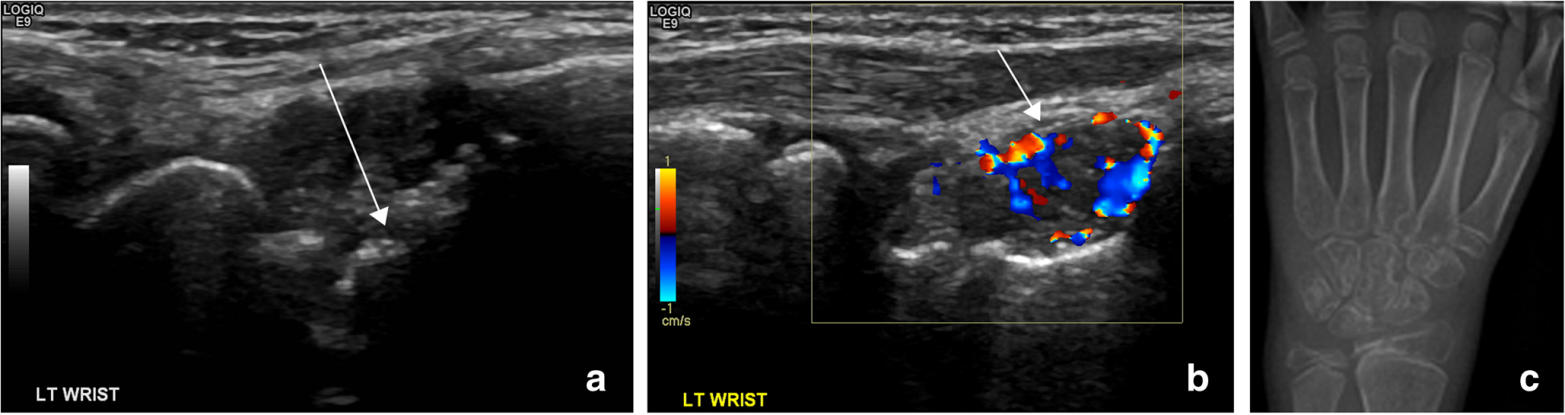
Ultrasound may depict both synovitis and bone destruction, particularly in small joints. a Ultrasound of the dorsal aspect of the wrist shows an irregular surface of the scaphoid (long arrow) suggestive of erosions. c There was thickened and hyperaemic synovium over the scaphoid, indicative of active inflammation. c Plain radiograph of the wrist confirmed squaring of the carpal bones, carpal crowding and a sclerotic, irregular bony surface
